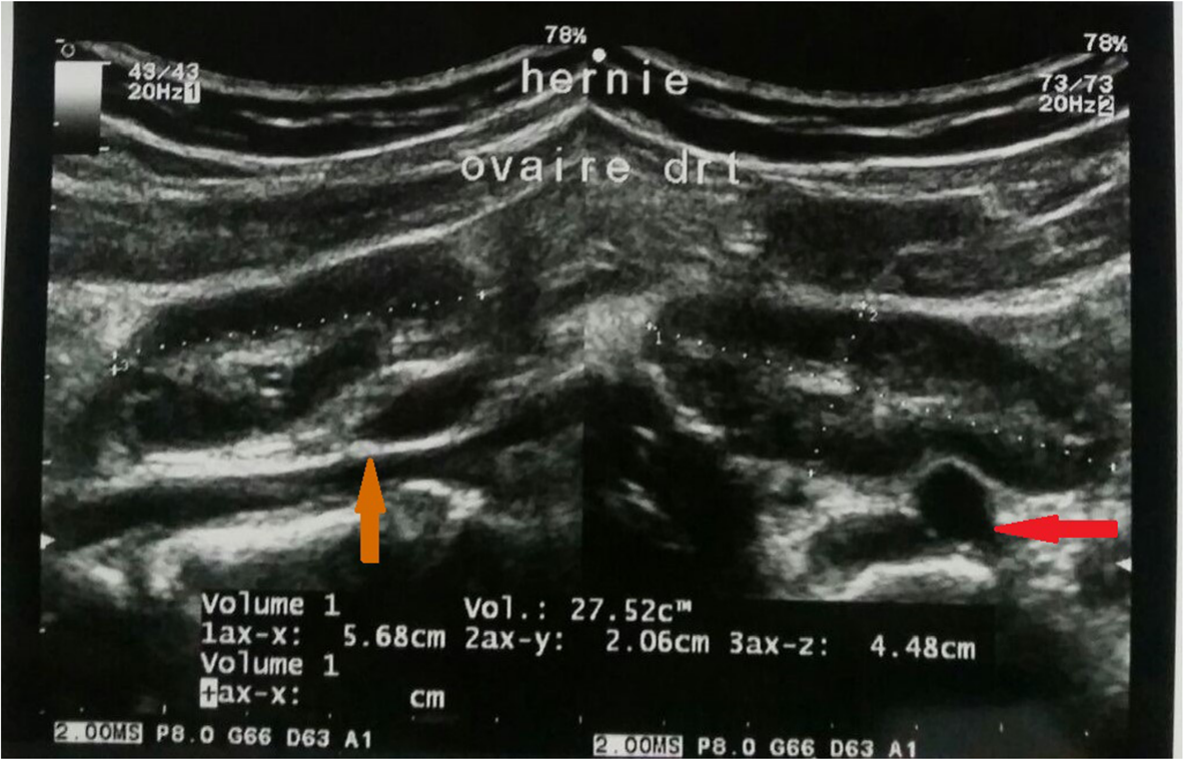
Pelvic ultrasound showing an edematous right ovary in longitudinal (left) and transverse (right) views. On the *left*, the ovary is superficial to the parietal peritoneum (*orange arrow*), and just beneath the subcutaneous tissues. On the *right*, the ovary is superficial to the external iliac artery (*red arrow*)
